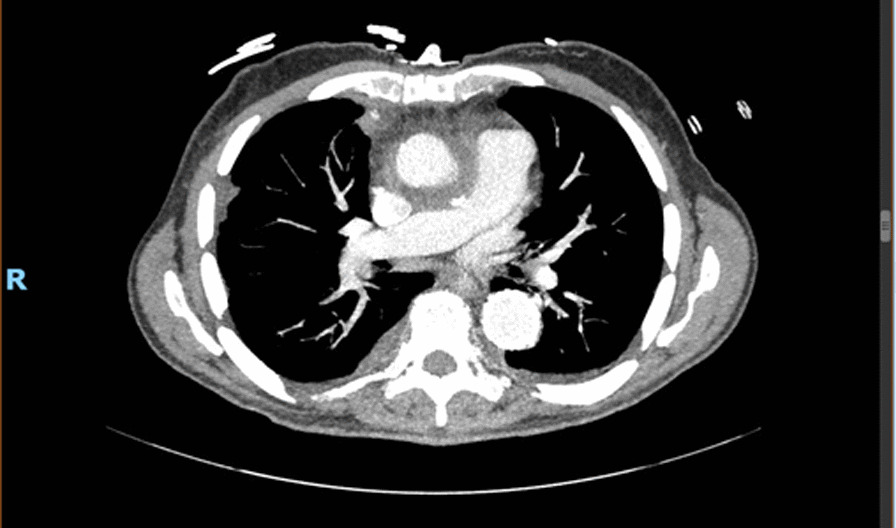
Computed tomography angiogram obtained 1 week postoperatively, demonstrating substantially decreased fluid around the ascending aorta
